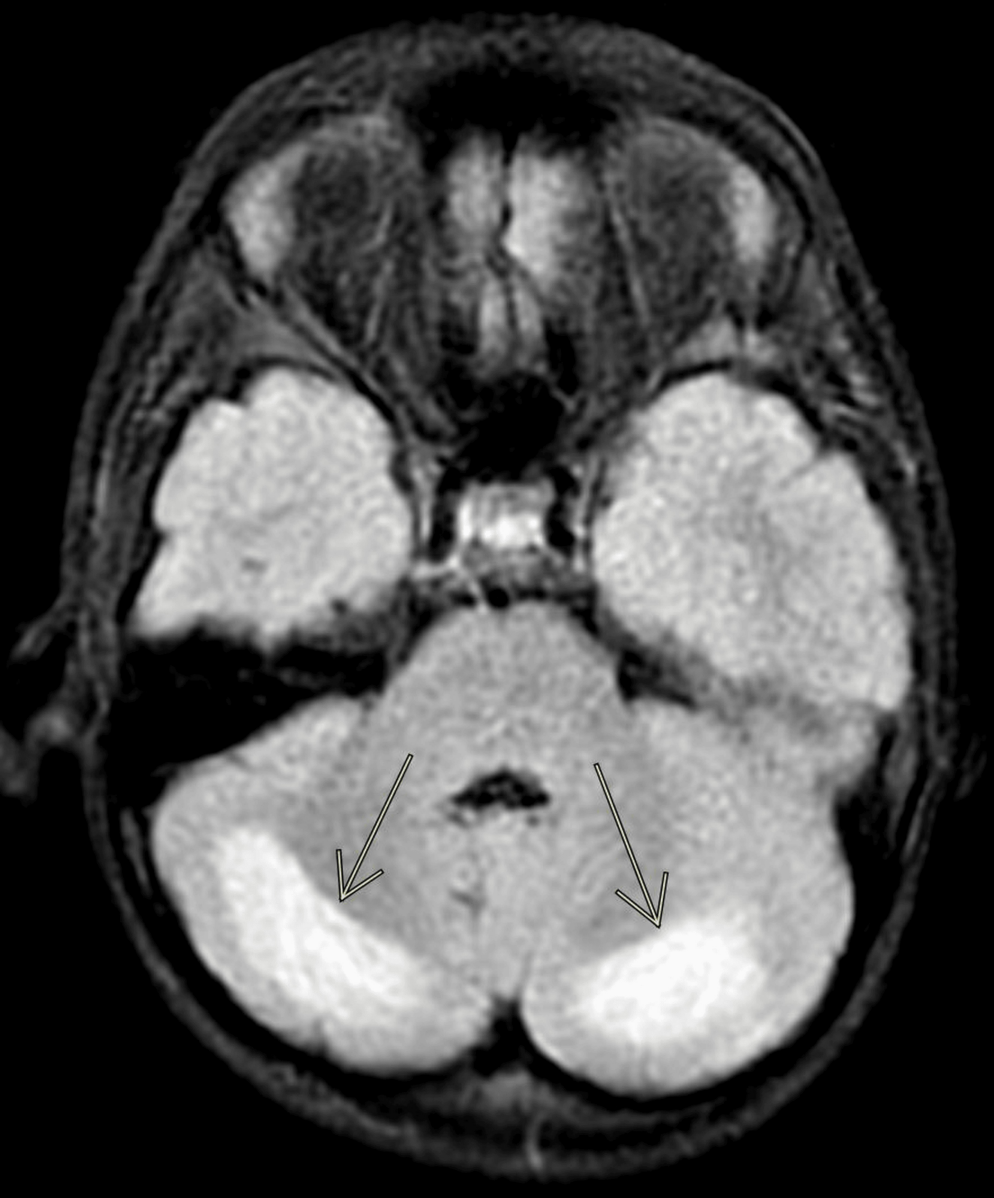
Brain MRI obtained on hospital day 3 Axial fluid-attenuated inversion recovery (FLAIR) shows a bilateral hyperintense signal in the cerebellar white matter.
